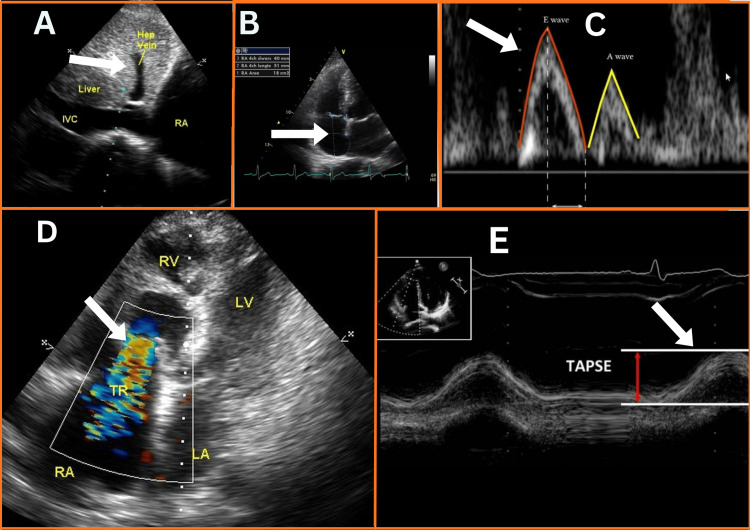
Representative echocardiographic measurements used in the assessment of pulmonary hypertension in chronic kidney disease. (A) Subcostal echocardiographic view showing the inferior vena cava and hepatic vein for assessment of right atrial pressure based on inferior vena cava diameter and inspiratory collapse.
